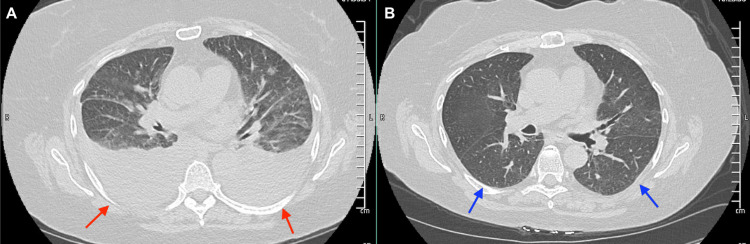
A: Computed tomography of the chest showing bilateral pleural effusions in a patient with renal failure due to PRL (red arrows). B: CT Chest showing resolution of bilateral pleural effusions after treatment (blue arrows). PRL: primary renal lymphoma
